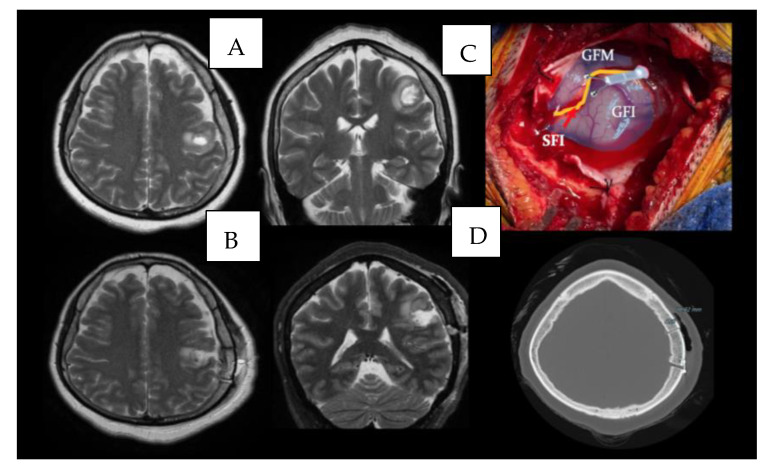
(A) Axial and coronal view of a preoperative T2 weighted MRI; (B) the postoperative axial and coronal view of a preoperative T2 weighted MRI that documents the GTR; (C) an intraoperative view of the cortical surface with the tracts highlighted; (D) the postoperative CT scan with bone algorithm that shows the small craniotomy.
